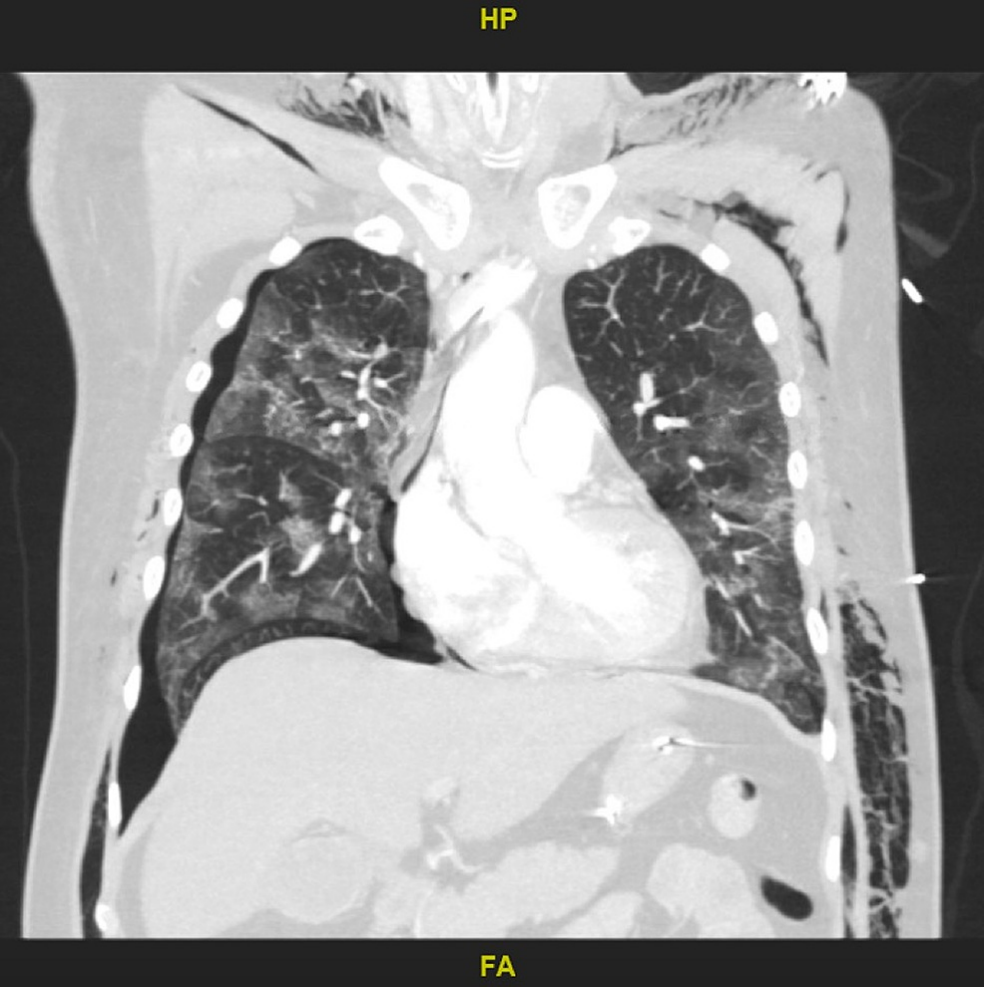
Coronal computed tomography showing extensive subcutaneous emphysema and a right-sided pneumothorax that required thoracostomy tube placement
